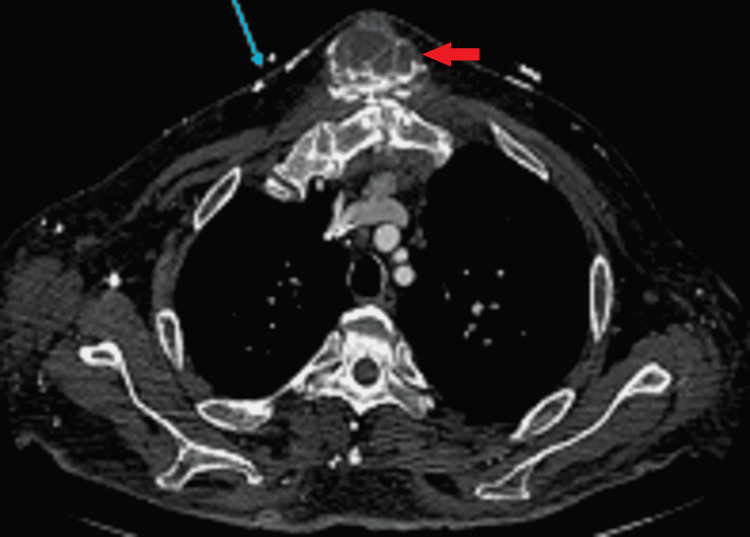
Initial axial contrast-enhanced conventional CT image demonstrating a multilobulated lesion in the soft tissues immediately superficial to sternotomy site with hyperattenuating layering material (red arrow) and reported as a hematoma with contrast material suggesting active bleed, especially given adjacent chest wall collaterals (blue arrow) from chronic right-sided central venous obstruction.
